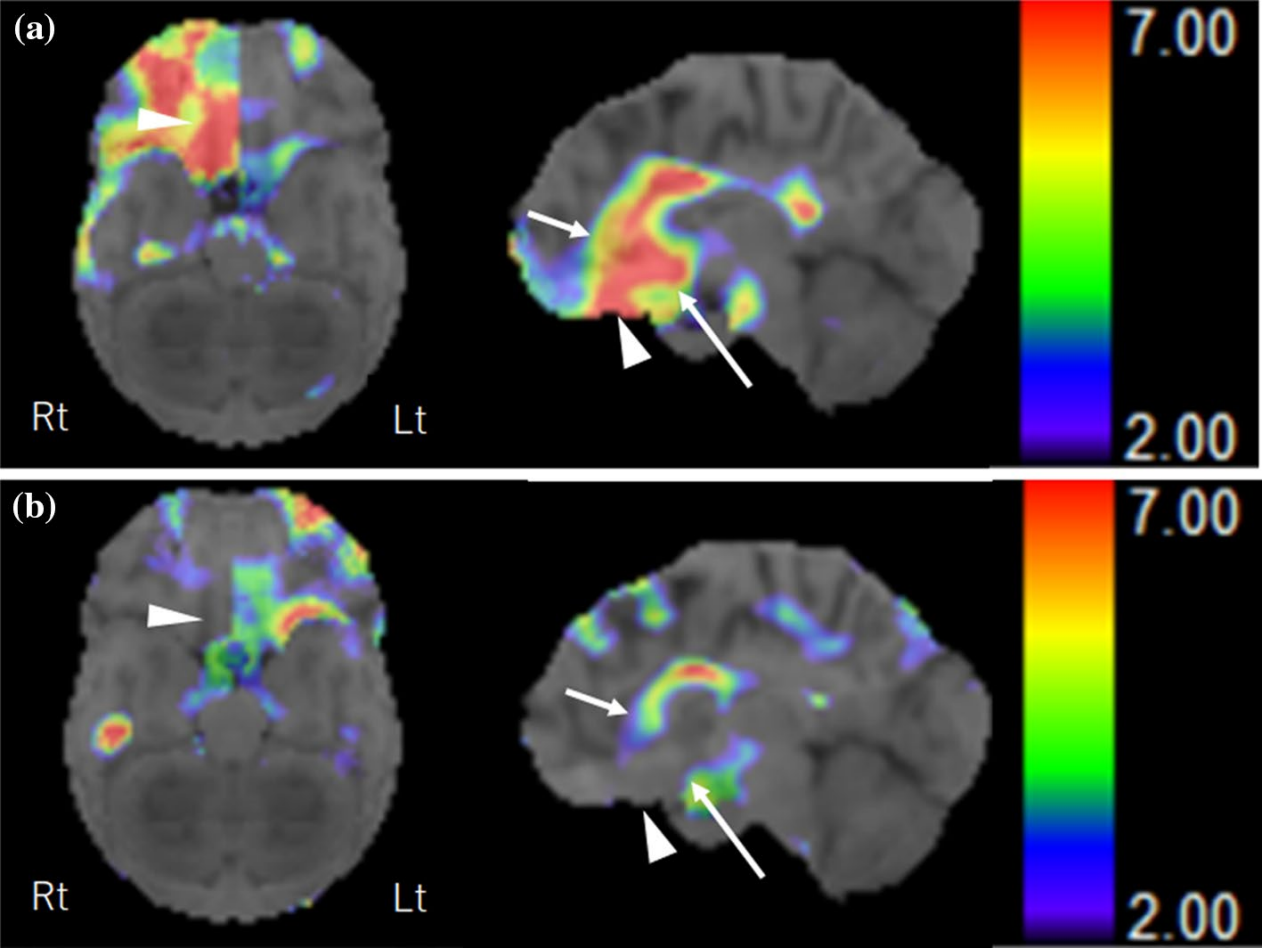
Stereotactic extraction estimation of decreased rCBF showing *Z* score. The left panel shows the inferior view and the right shows the medial view of the right hemisphere. The color scale bar shows the *Z* score ranging from 2 to 7. a Patient 1: a 52-year-old man presented with ruptured aneurysm of ACoA. Surgical clipping for the aneurysm was performed on the day of onset. Formal neuropsychological examination and SPECT were performed 2 months after the treatment of aneurysm. High Severity of decreased rCBF was observed in the subcallosal gyrus (5.13, arrow), anterior cingulate gyrus (5.78, short arrow), and rectal gyrus (7.99, arrowhead) on the right. All three MQs on the WMS-R were severely decreased (Verbal MQ: 62, Visual MQ: 69, and General MQ: 58). b Patient 4: a 45-year-old man presented with ruptured aneurysm of ACoA. Surgical trapping of ACoA for the ruptured ACoA aneurysm was performed the day of onset. Formal neuropsychological examination and SPECT were performed 3 months after the treatment of aneurysm. Low Severity of decreased rCBF was observed in the subcallosal gyrus (3.67, arrow), anterior cingulate gyrus (3.42, short arrow), and rectal gyrus (2.33, arrowhead) on the right. All three MQs on the WMS-R were preserved (Verbal MQ: 91, Visual MQ: 98, and General MQ: 92)
